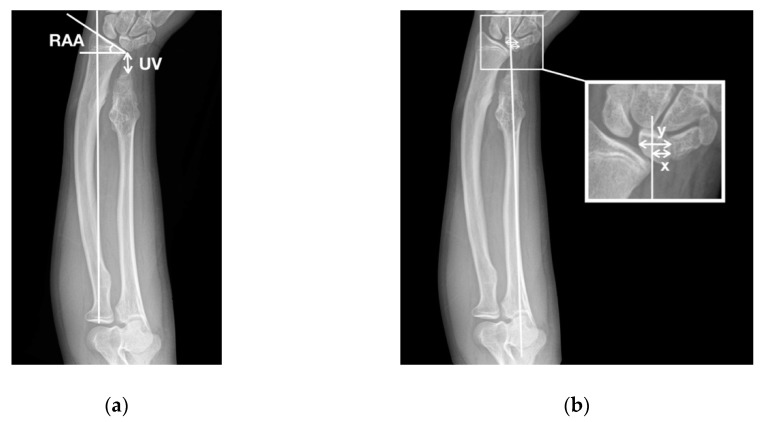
Radiographic parameters. (a) Ulnar variance (UV): the distance between the distal ulnar epiphysis and the ulnar side of the distal radial epiphysis. Radial articular angle (RAA): the angle defined between a line along the radial articular surface and a line perpendicular to the line drawn from the center of the radial head to the radial border of the distal radius. The normal value is 15–30°; (b) carpal slip (CS): the percentage of the lunate that falls on the ulnar side, with a reference line drawn from middle of the olecranon to the ulnar border of the radial epiphysis (*x*/*y* × 100%). The normal value is 50%.
